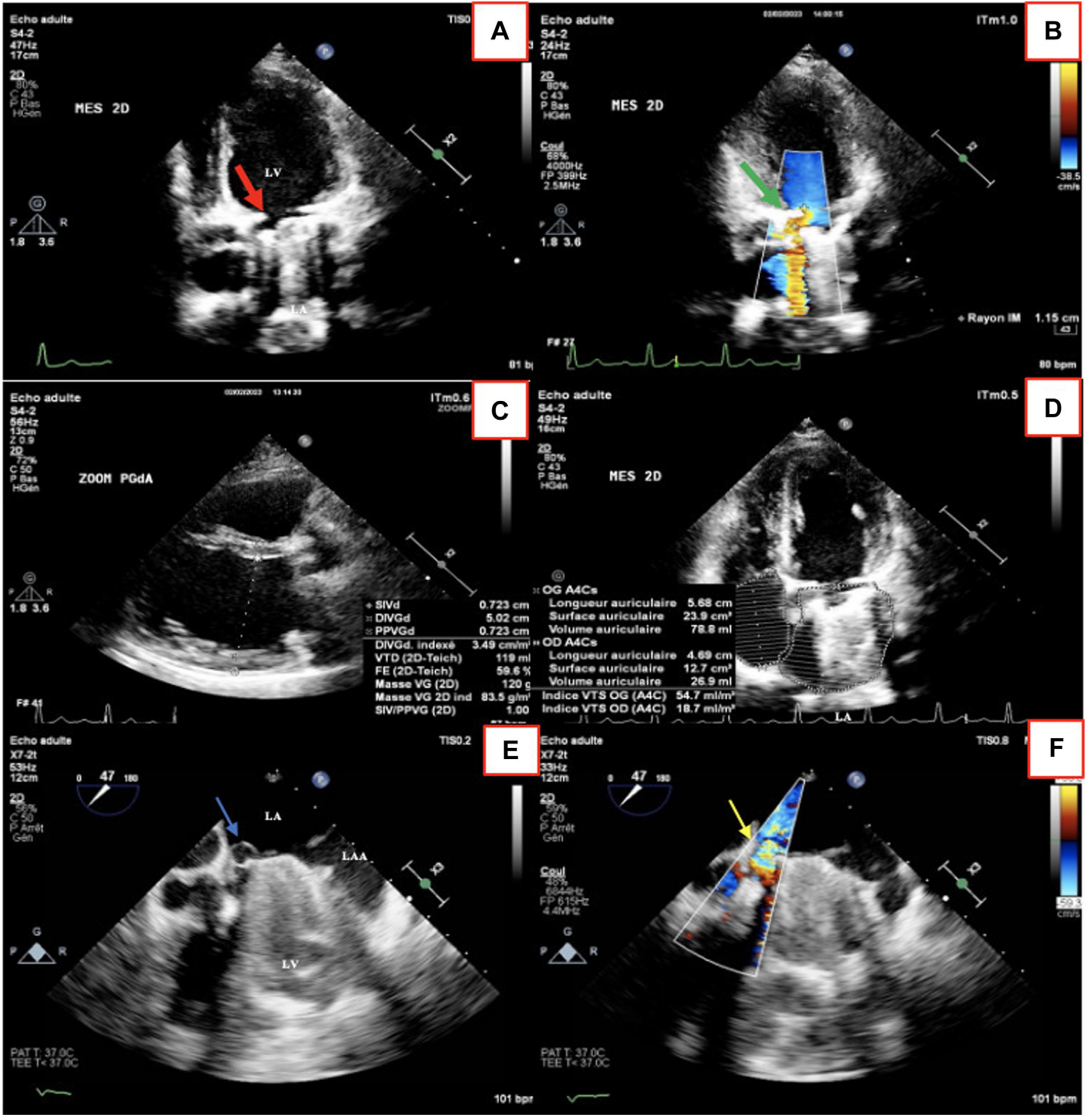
Multimodal echocardiographic assessment. (A) Transthoracic echocardiography (TTE) modified 4-chamber view, showing a mechanical bileaflet prosthesis in the mitral position with partial prosthesis detachment in the septal region (red arrow). (B) TTE modified 4-chamber view with color Doppler analysis, showing a severe paravalvular regurgitation jet (green arrow). (C) TTE parasternal long-axis view, showing a dilated left ventricle secondary to severe mitral regurgitation. (D) TTE 4-chamber view, showing a dilated left atrium secondary to severe mitral regurgitation. (E) Transesophageal echocardiography (TEE) modified 4-chamber mid-esophageal view, revealing a partial dehiscence of the mitral prosthesis, with mobile elements bent around the exterior of the annulus and protruding into the left atrium, suggesting the presence of vegetations or suture threads (blue arrow). (F) TEE with color Doppler analysis, showing a severe paravalvular regurgitation jet (yellow arrow). LA, left atrium; LAA, left atrial appendage; LV, left ventricle.
